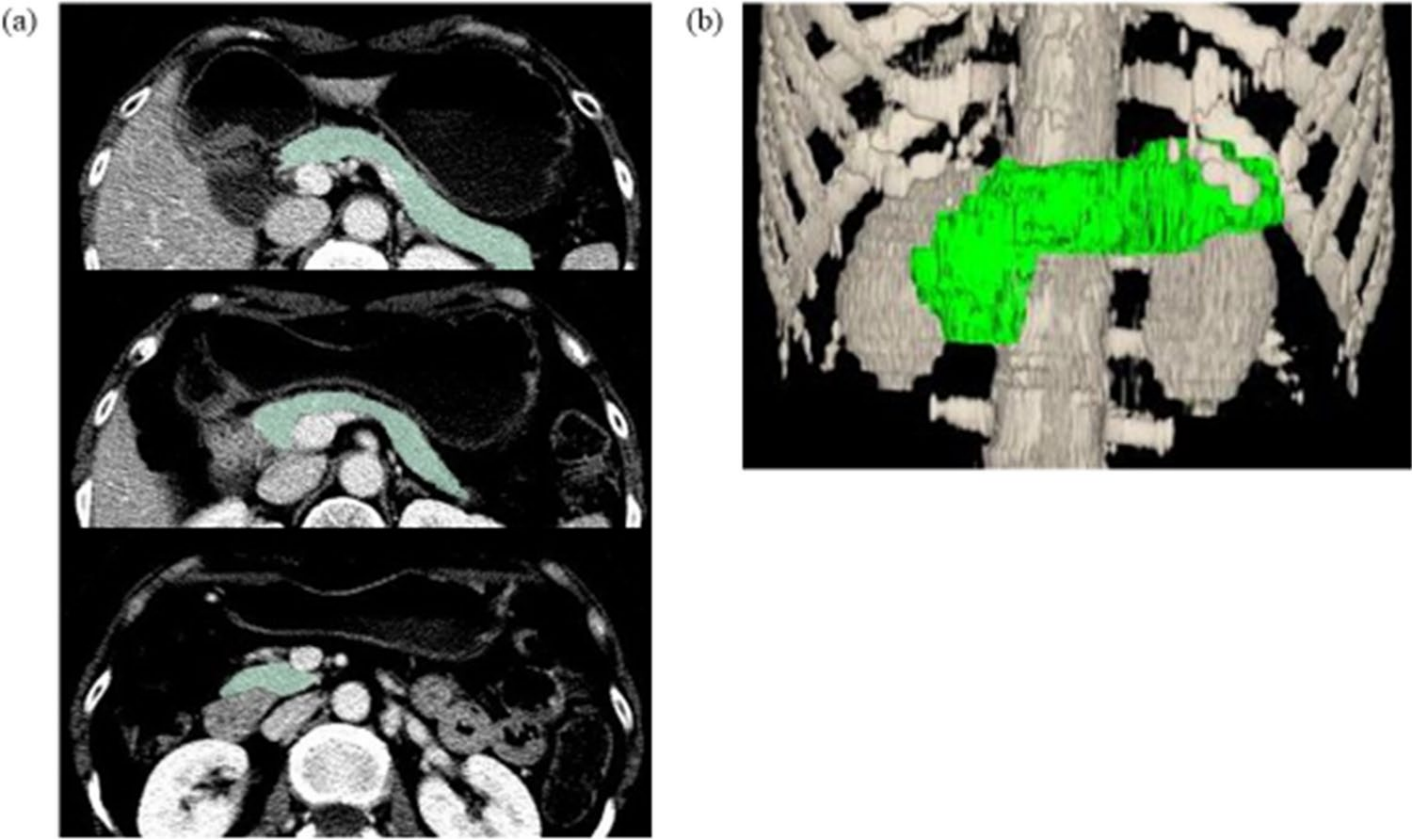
An example of CT volumetry when measuring the pancreas volume. a A cross-sectional image of the pancreas, b a constructed 3D image of the pancreas
